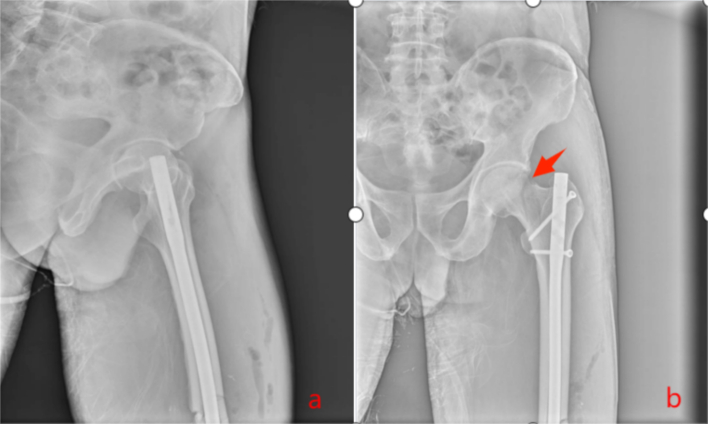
Postoperative day 2 radiographs revealed an incomplete subcapital femoral neck fracture (Pauwels type III).
